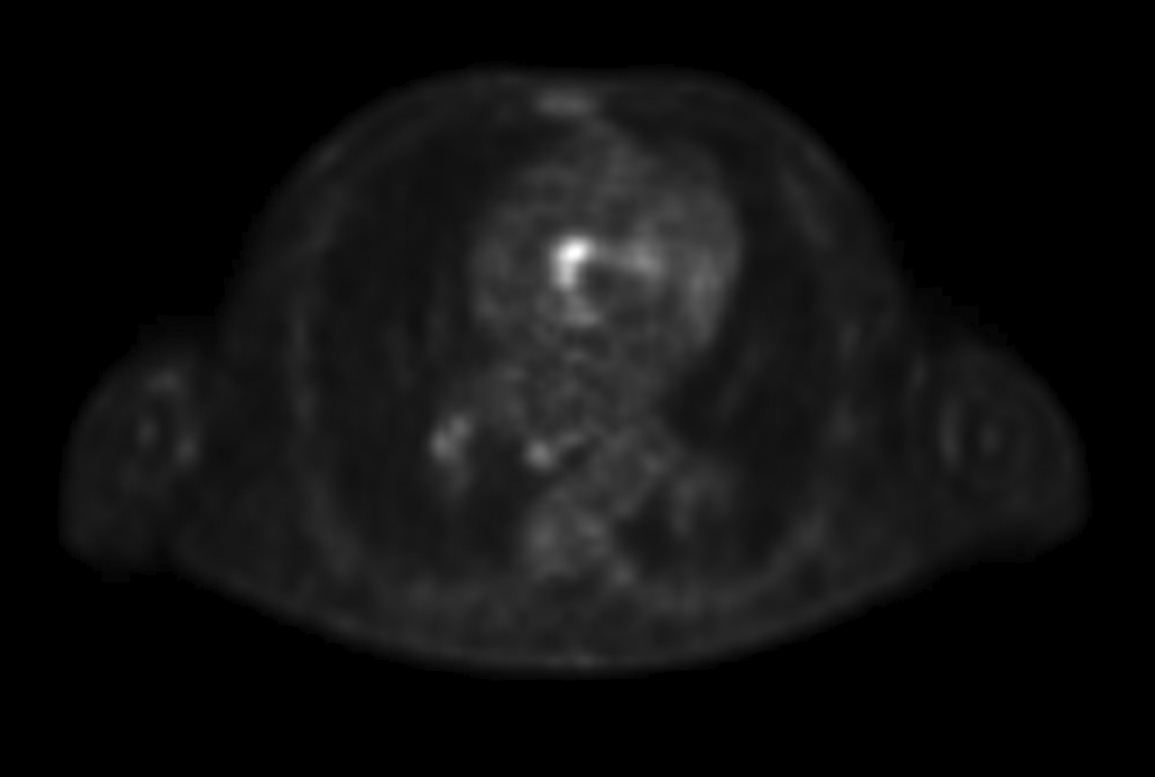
Fluorodeoxyglucose‐positron emission tomography scan demonstrating low grade uptake in the heart in vicinity of aortic‐mitral valve rings. At this time, the transesophageal echocardiogram did not demonstrate any valvular vegetations.
